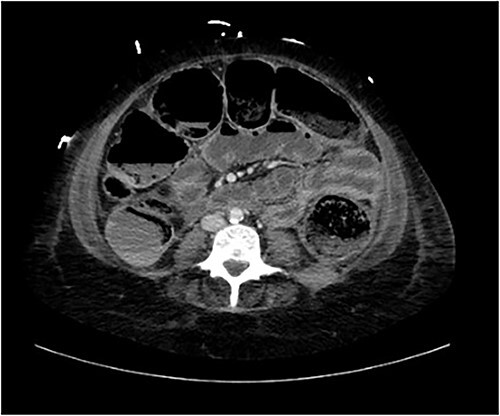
Axial view of CT abdomen demonstrating normal large bowel wall enhancement with faecal loading.
